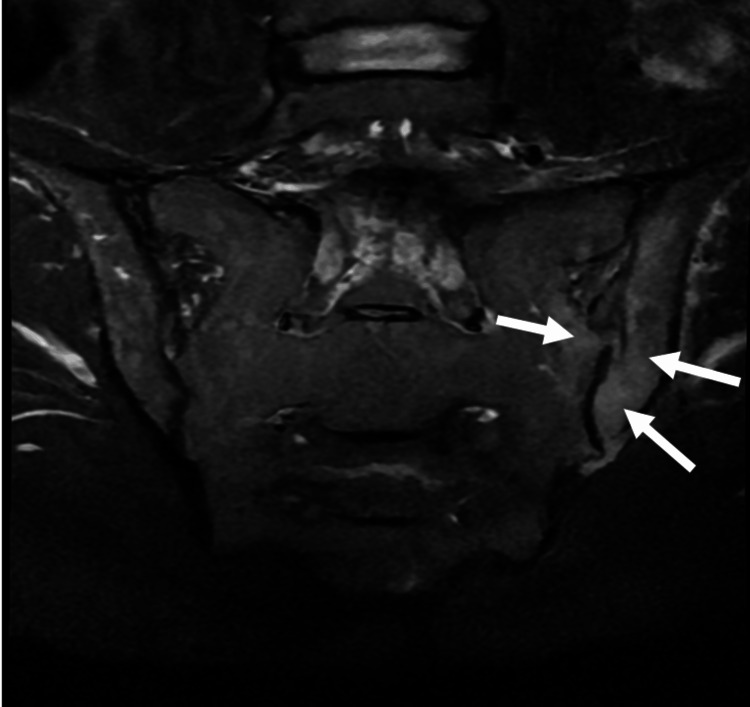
Coronal sacrum and coccyx MRI with contrast, showing an increased T2 signal within the left SI joint and adjacent osseous structures (white arrows) MRI: magnetic resonance imaging; SI: sacroiliac
